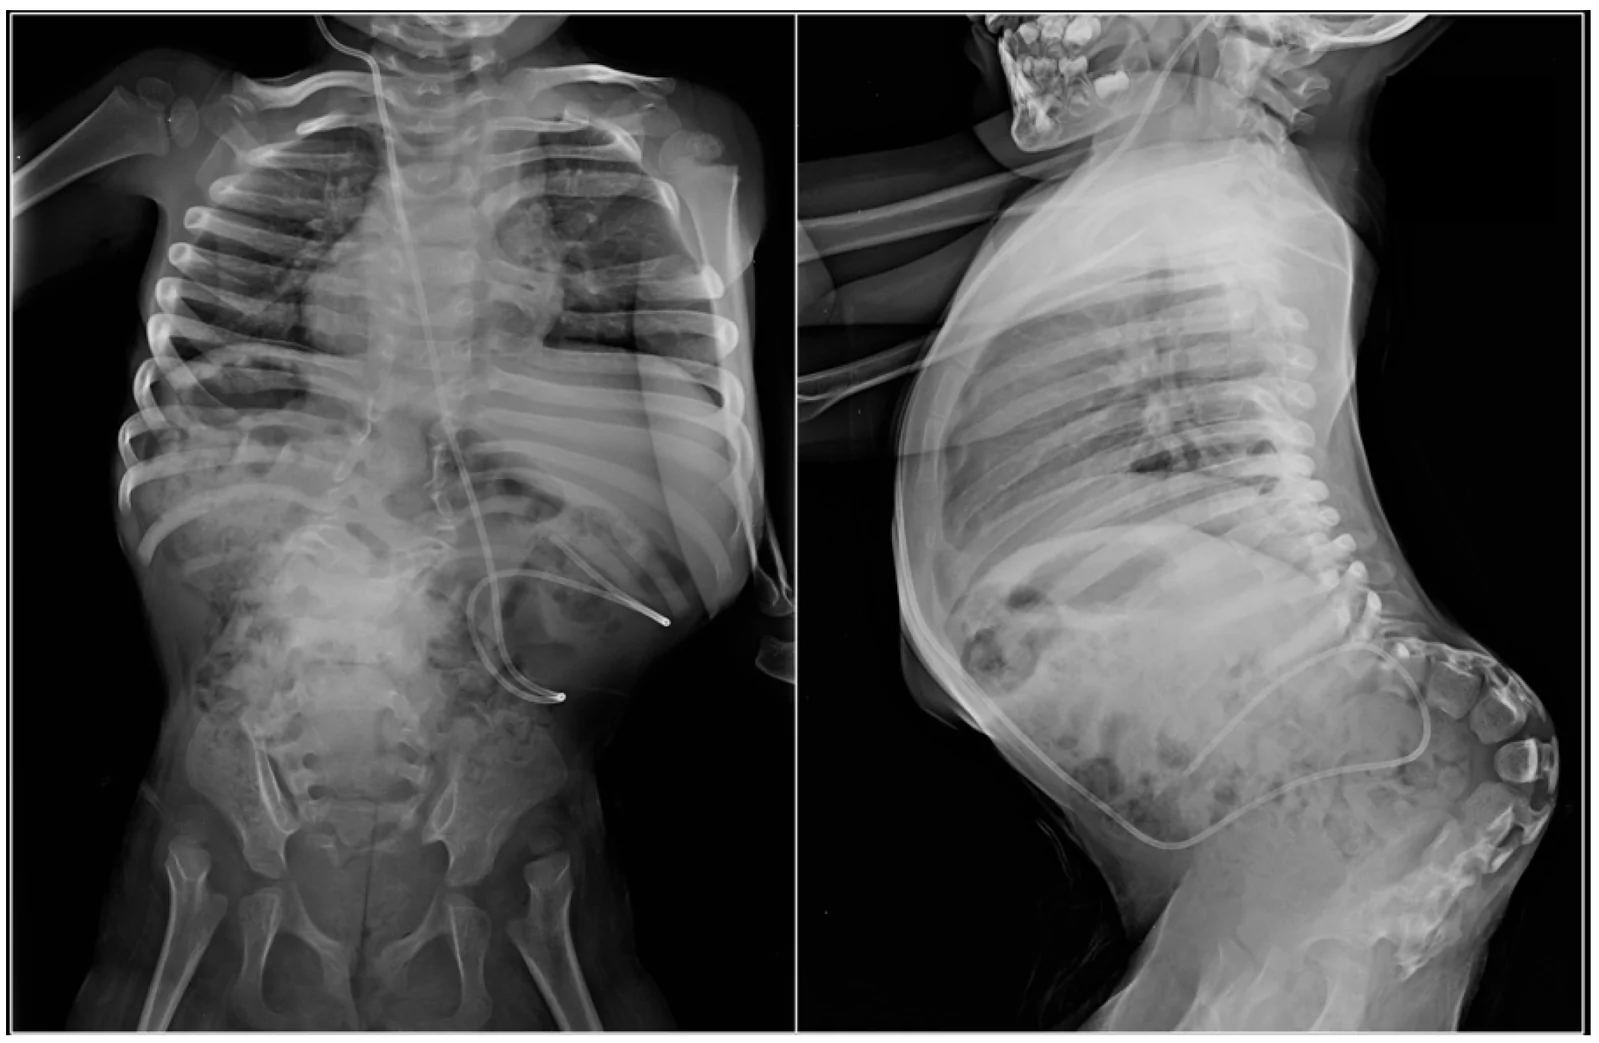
Anteroposterior and lateral radiographs of a patient with Jarcho–Levin syndrome show multiple vertebral segmentation anomalies, rib abnormalities, thoracic deformity, pelvic obliquity, and bilateral hip dislocation.
